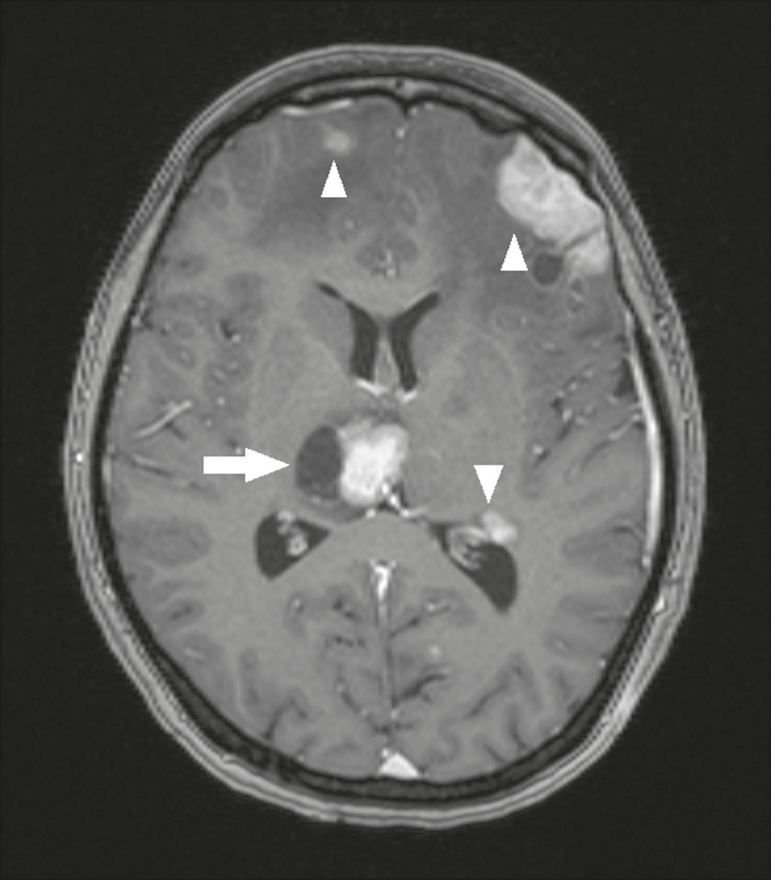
Metastases in a patient with clear cell renal cell carcinoma. Contrast- enhanced axial T1-weighted MRI sequence showing an expansile lesion, with contrast uptake, in the right thalamus, as well as other multiple lesions on both sides (arrowheads).
